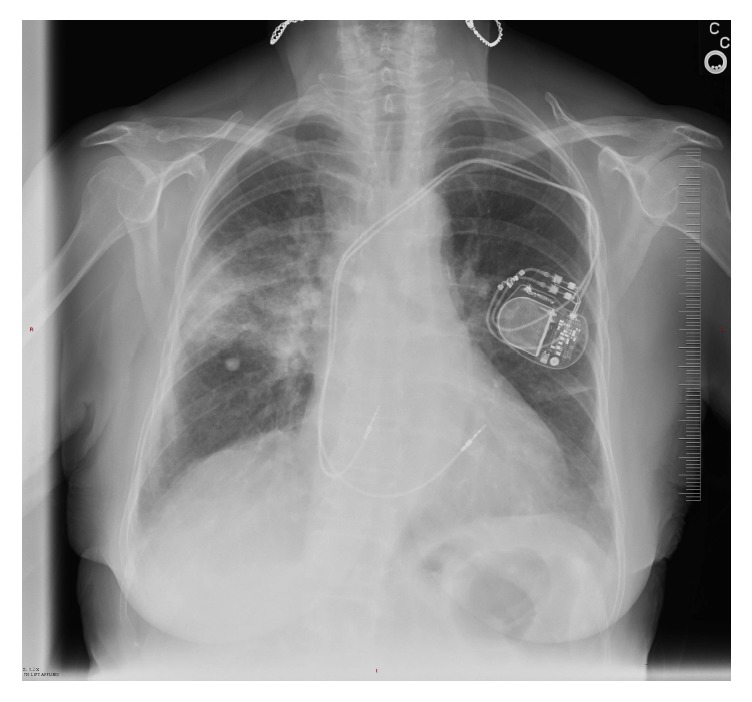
A chest X-ray showing the development of lung infiltrates in and around the treated right lung mass occurring one month following completion of SBRT.
